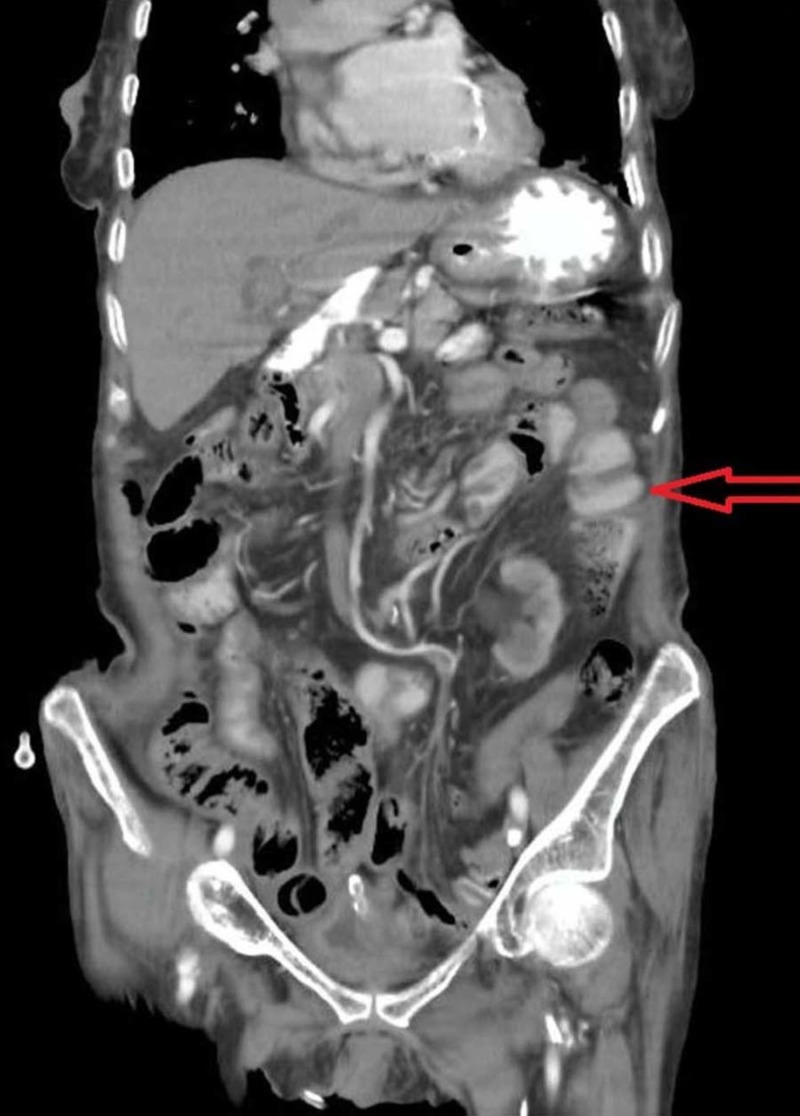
Computed tomography (CT) scan of the chest and abdomen revealing dilated bowel loops
